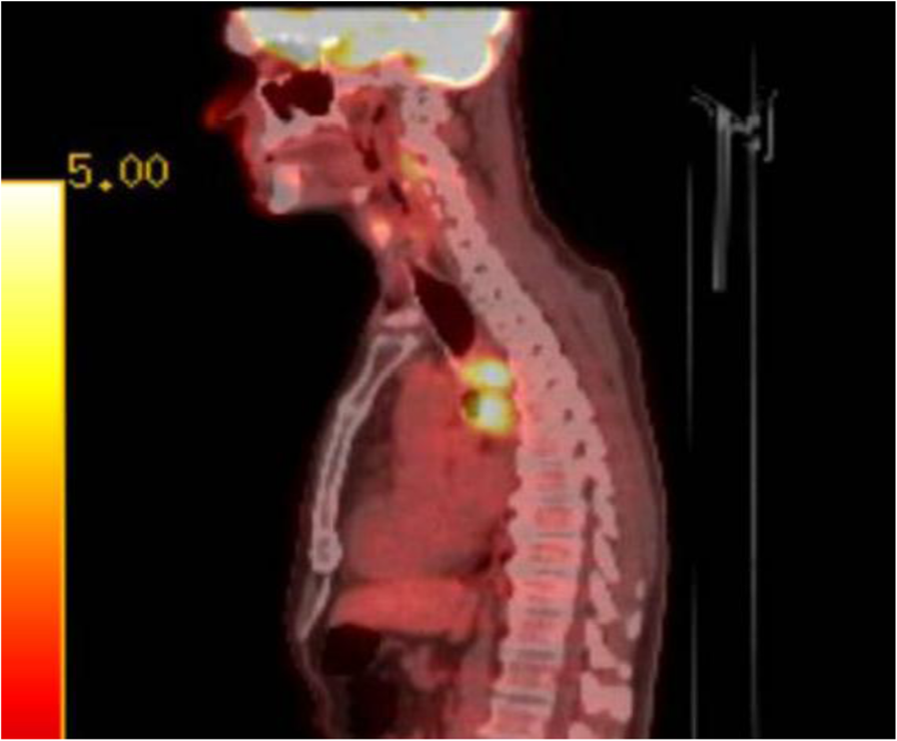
Sagittal positron emission tomography-computed tomography imaging demonstrating a hypermetabolic lesion in the posterior mediastinum with increased levels in the midesophagus.
